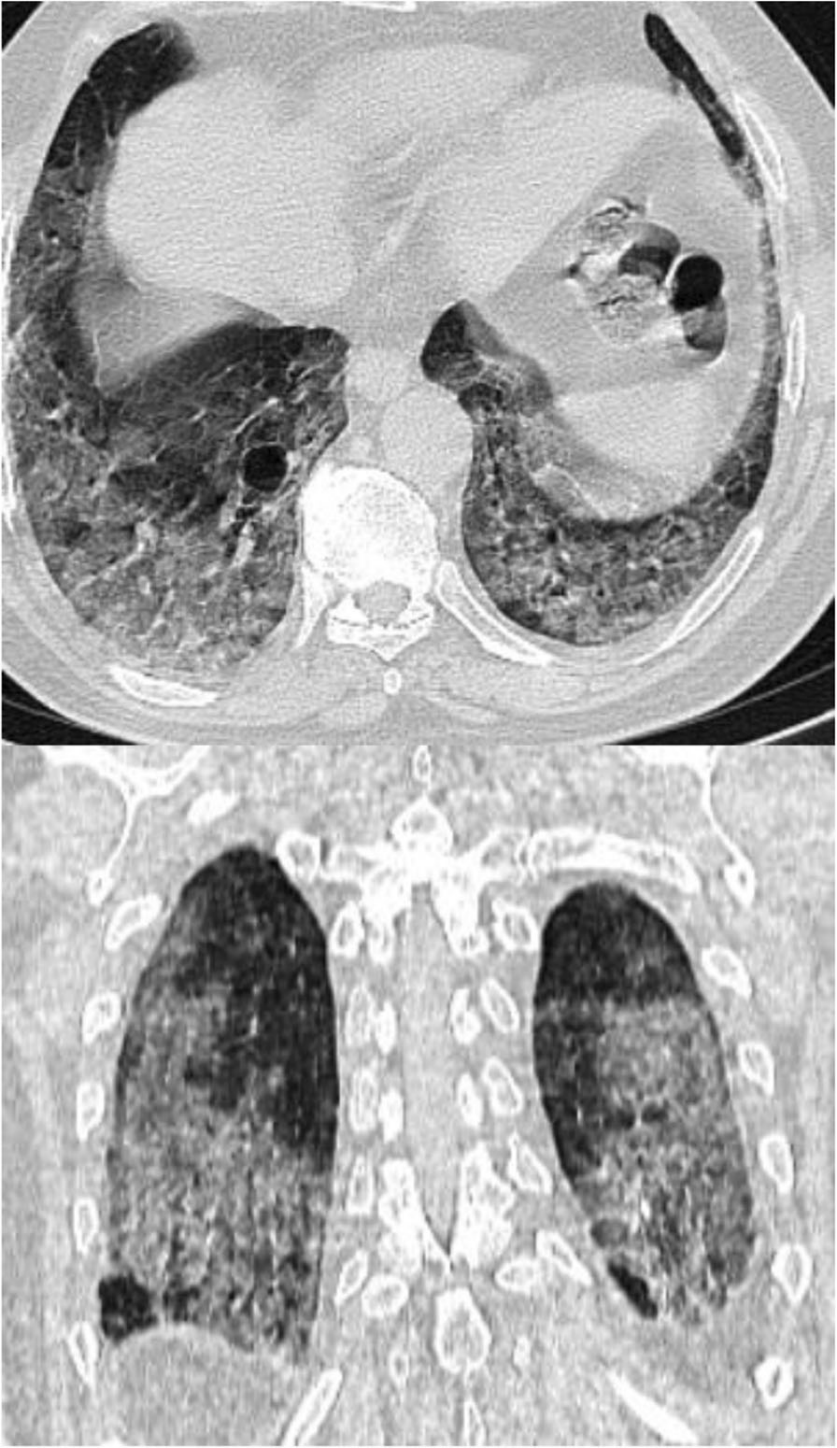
Axial and coronal CT images of patient with COVID-19, 10 days after the onset of symptoms, showing extensive ground glass infiltration with crazy paving infiltration. The appearance of crazy paving appearance indicates a progressive disease
